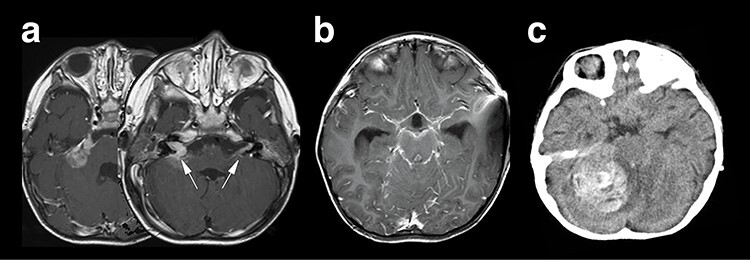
Neuroradiological representation of the lesions, T1WI showed a homogeneously enhanced lesion at the cerebellopontine angle spreading to the bilateral auditory canal (white arrows) (a), and a widespread leptomeningeal enhancement was encasing the brainstem (b). Unfortunately, the patient’s condition suddenly deteriorated, and the CT image revealed a massive intra-tumoral hemorrhage compressing the brainstem (c).
